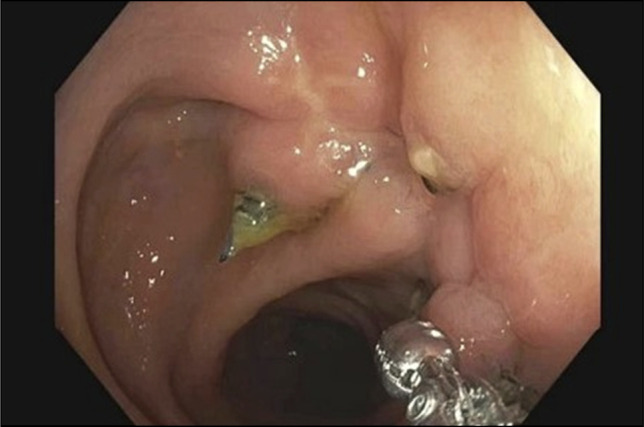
Surveillance endoscopic ultrasound at 6 months follow-up revealing well-healed resection site with healthy-appearing mucosa.
